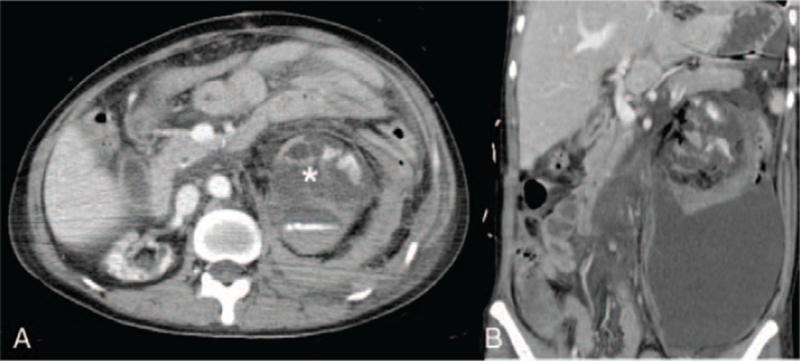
(A) Contrast extravasation of the left renal artery in computed tomography (transverse section). Asterisk: Contrast extravasation of the left renal artery. (B) Contrast extravasation of the left renal artery with massive left renal hematoma in computed tomography (coronal section).
